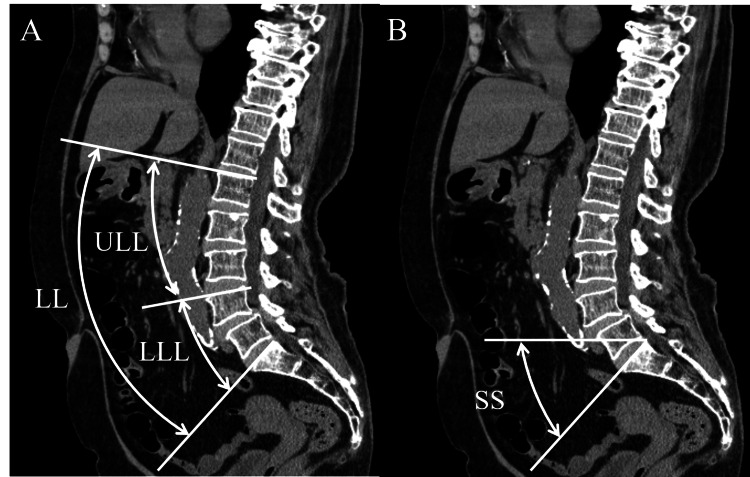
Measurement of sagittal lumbopelvic parameters (A) Using sagittal reconstructed CT images, lumbar lordosis ([LL] angle between the superior endplates of L1 and S1), upper lumbar lordosis ([ULL] angle between the superior endplates of L1 and L4), and lower lumbar lordosis ([LLL] angle between the superior endplates of L4 and S1) were examined. (B) The sacral slope ([SS] angle between the superior endplate of S1 and the horizontal plane) was also examined.
